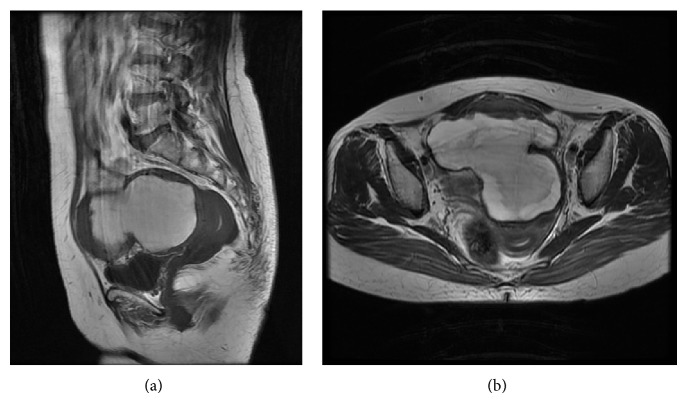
Magnetic resonance imaging of the pelvis. Lobulated masses in the pelvic region (the largest being 10 cm in diameter) that contained a hemorrhagic component and a solid part at the center that exhibited a low intensity on T2-weighted images are shown in both (a) sagittal (T1WI) and (b) transverse (T2WI) sections.
